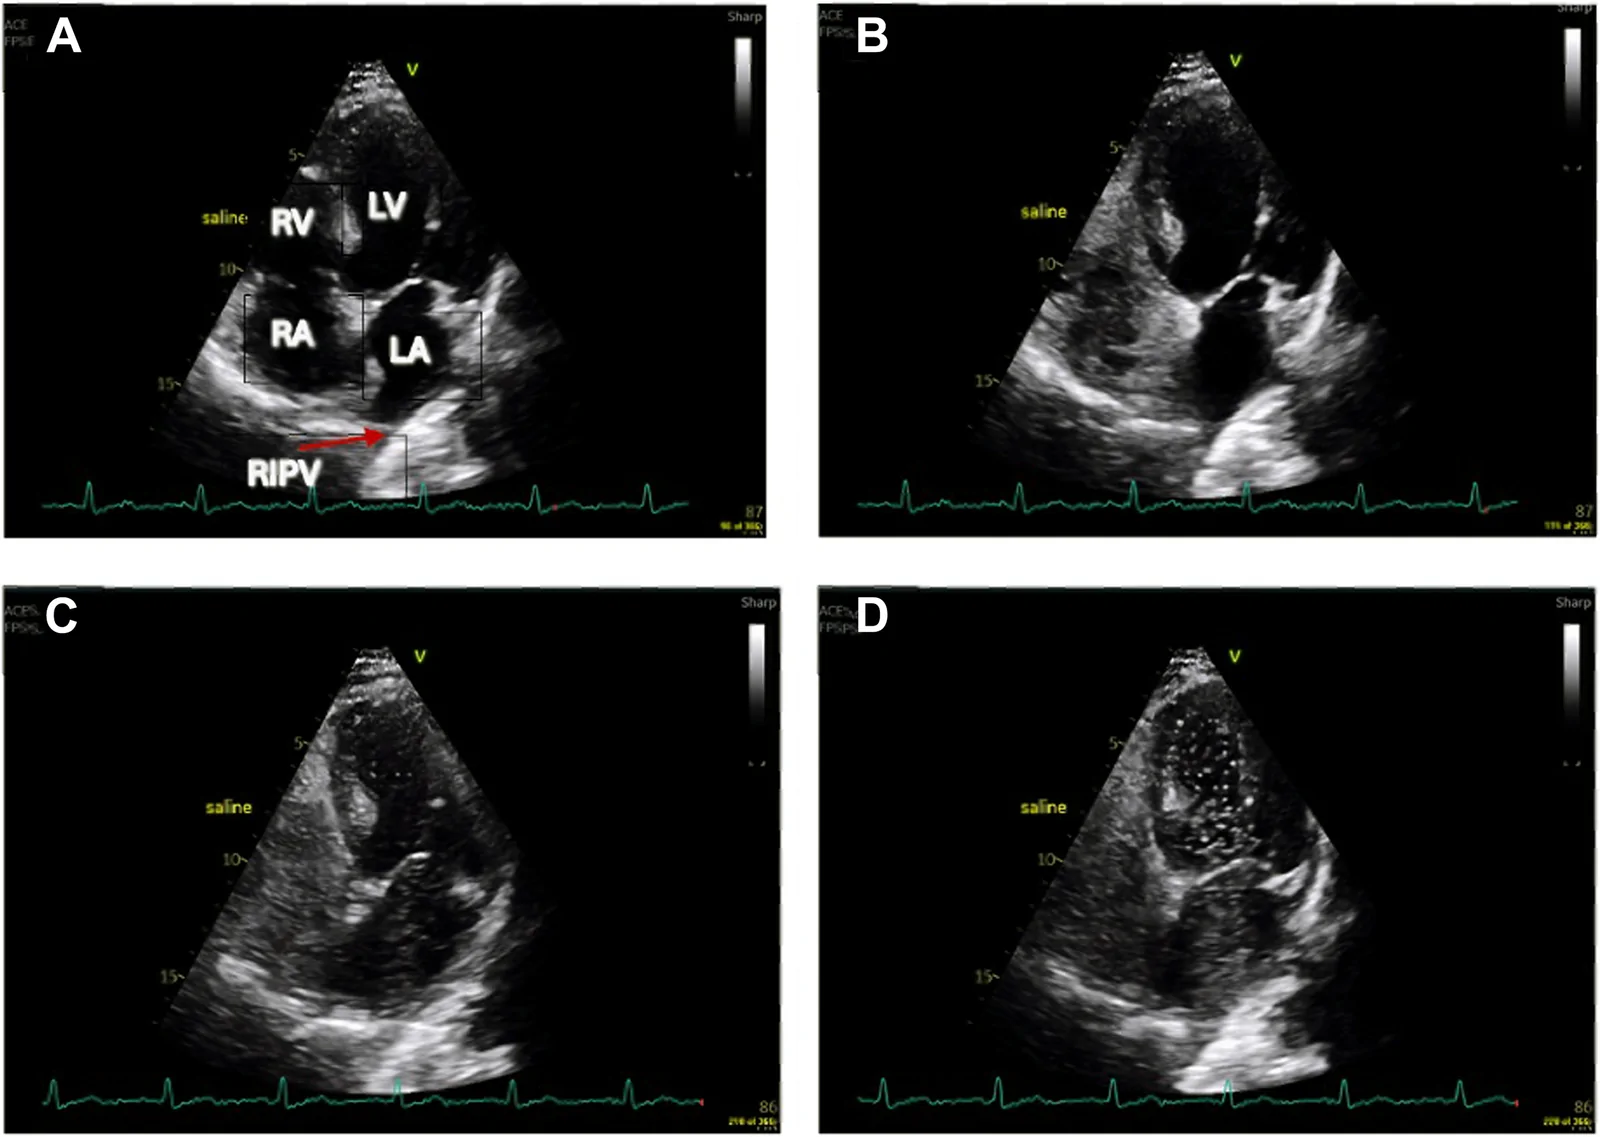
Sequential 4-chamber transthoracic echocardiography images of the patient with angulation for the pulmonary veins. A, Before saline echocontrast injection demonstrating anatomy. B, Beat 1 after injection appearance of echocontrast in the right side of the heart. C, Beat 4 after injection echocontrast appearing in the left atrium via the pulmonary vein. D, Beat 5 after injection opacification of the left side of the heart with endocardial definition suggesting significant shunt. LA = left atrium; LV = left ventricle; RA = right atrium; RIPV = right inferior pulmonary vein; RV = right ventricle.
